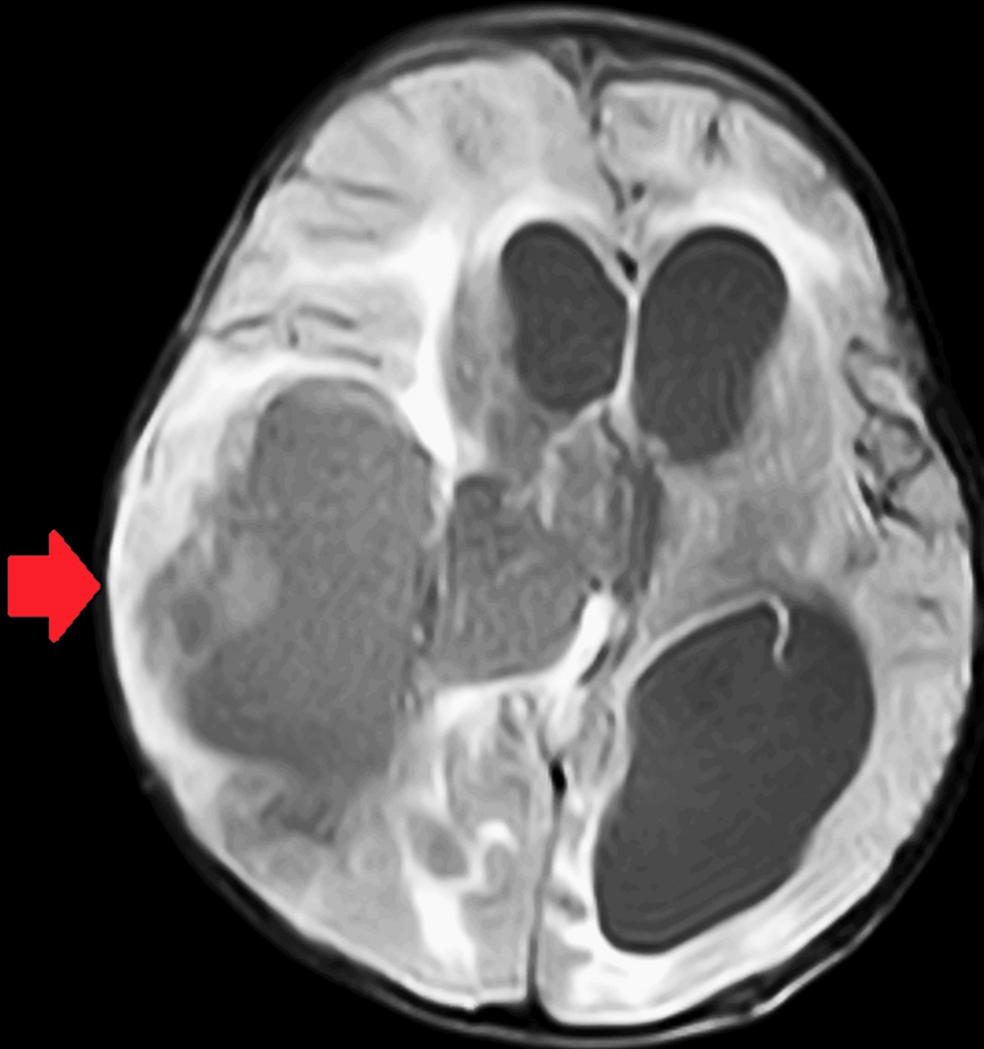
A large cystic lesion with an eccentric fluid-attenuated inversion recovery (FLAIR) heterogeneous component in the right fronto-parietal lobes, measuring 7.7 × 5.8 × 8.5 cm
